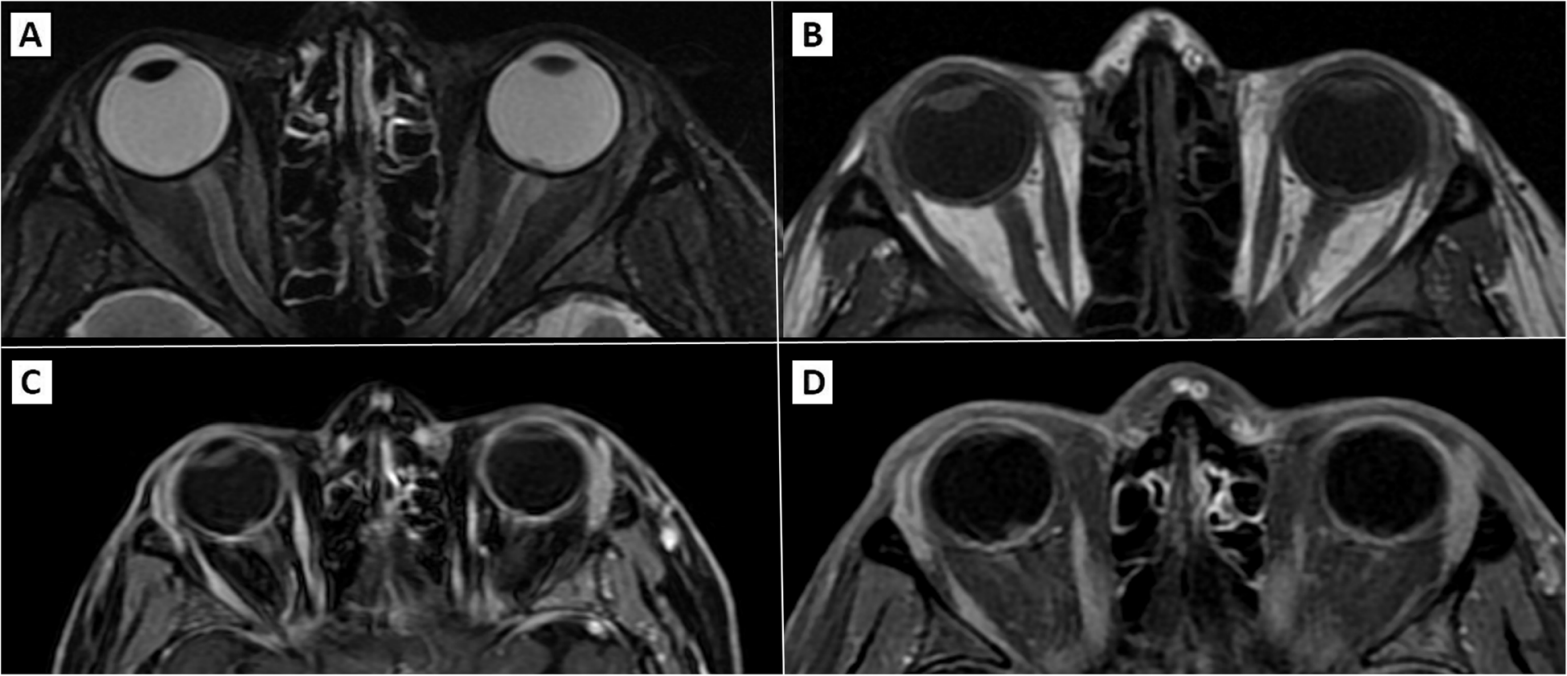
Left and right orbits MRI scans with presence of small nodules on both optic disks, especially on the right eye, which was compatible with bilateral leukemic infiltration. Optic nerves, chiasma, and other neurological segments were unaffected. (A): T2-weighted, a small nodule is best evidenced on the left eye; (B): T1-weighted without contrast and fat suppression, evidencing no impairment of optic nerves; C/D: T2-weighted with gadolinium contrast, small nodules of the left eye are highlighted by the gadolinium contrast
